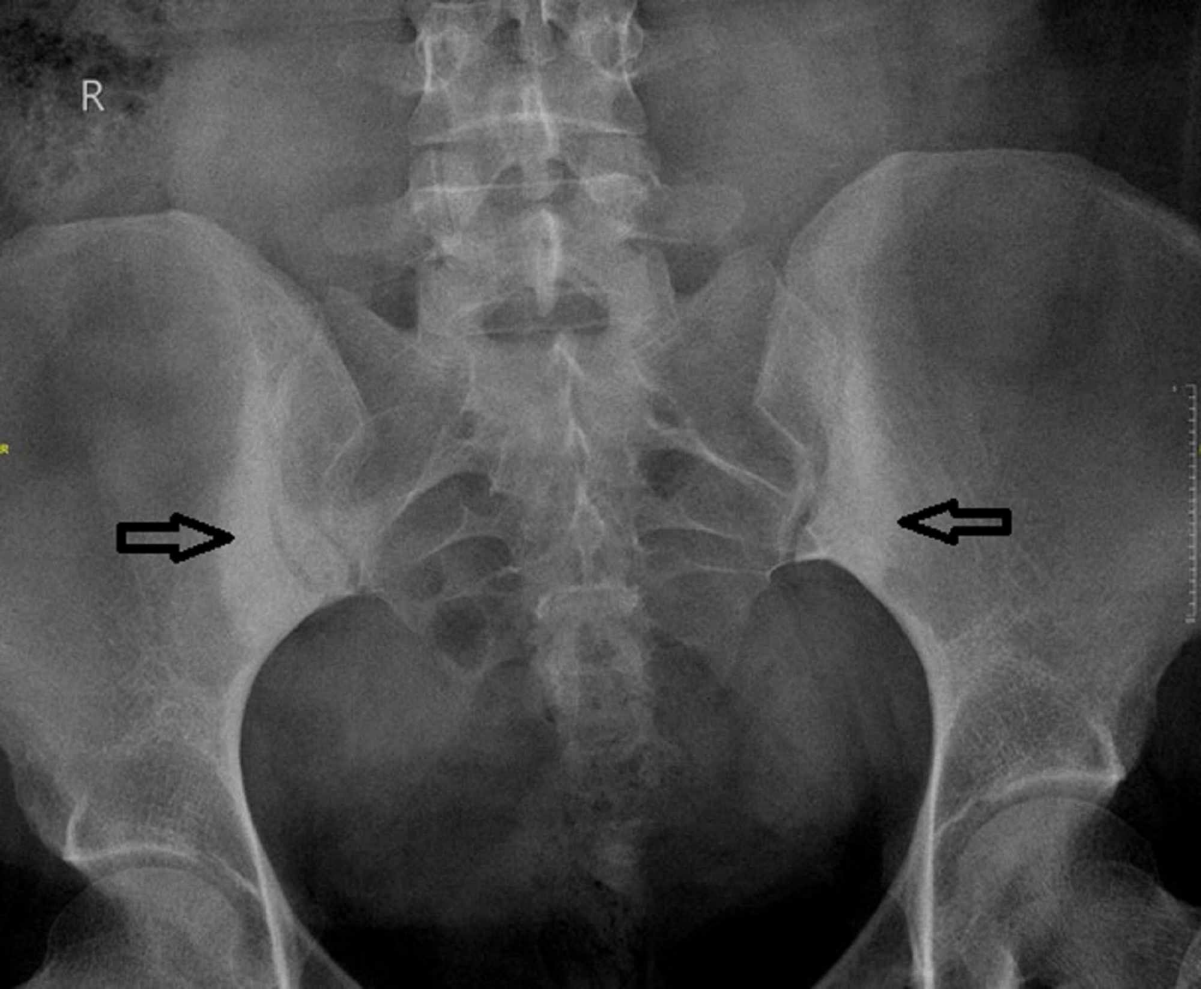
X-ray of the sacroiliac joint Anterior-posterior view showing significant sclerosis at the iliac border of the sacroiliac joints.
